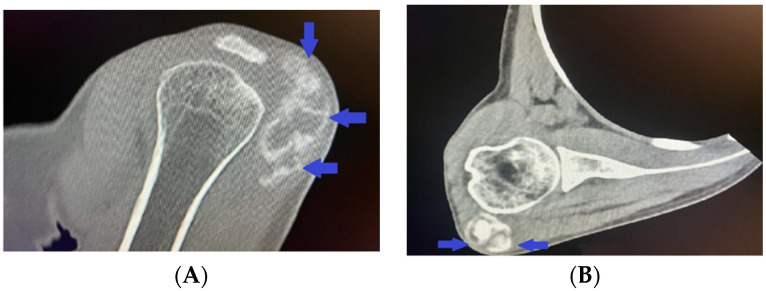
(A,B) Computed tomography with 3D reconstruction showing cloud-like lesion with soft-tissue and calcium-equivalent densitometric density in the right deltoid muscle lateral to the right humeral head and not involving adjacent bones and structures.
